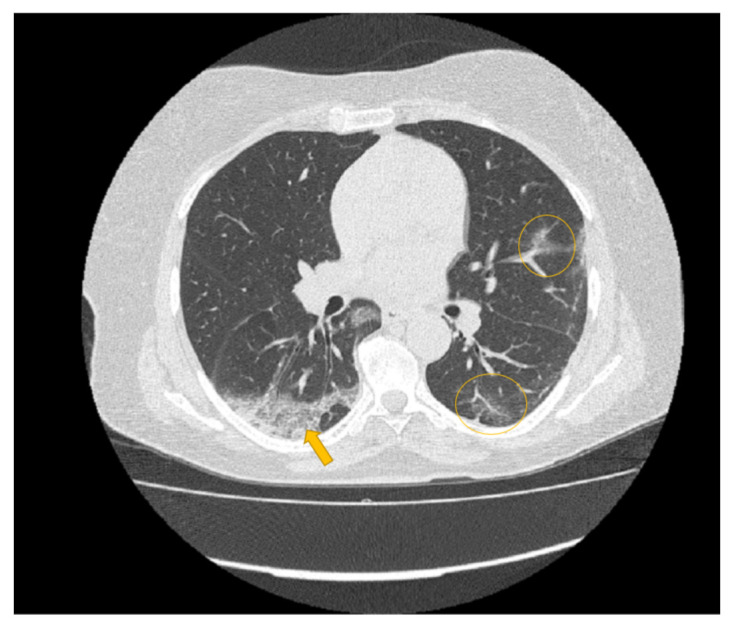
HRCT scan of a COVID-19 patient. The image revealed a lesion in the form of “crazy paving” seen in the right lung lower lobe with peripheral distribution (yellow arrow) and ground glass opacity seen in the left lower lobe (yellow circles) peripheral and central distribution.
